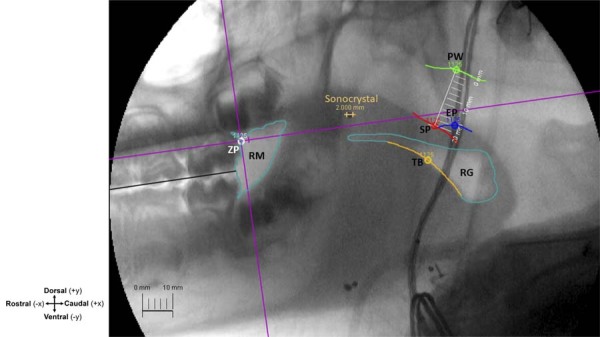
Preset coordinate system and tracing points on each oropharyngeal structure. The X-axis is defined as a line extending across the alveolar ridge of the upper molars, parallel to the occlusal plane (arrow), and the Y-axis is perpendicular to the X-axis, passing through the distal surface of the last upper molar. The intersection between the X- and Y-axes is used as the zero point (ZP). Digitized markers (small circles) for the soft palate (SP), tongue base (TB), epiglottis (EP), and pharyngeal wall (PW) were traced during inspiration and expiration in the coordinate system. The system was calibrated using the standard 2-mm size of the ultrasonic crystals implanted before baseline recordings. Blue lines indicate areas of the retromolar (RM) and retroglossal (RG) regions. The velopharyngeal width was measured as the linear distance between the points of PW and SP (ruler). The double-arrowed cross (lower left) indicates anatomical orientations for all structural tracing. Scale: 10mm.
